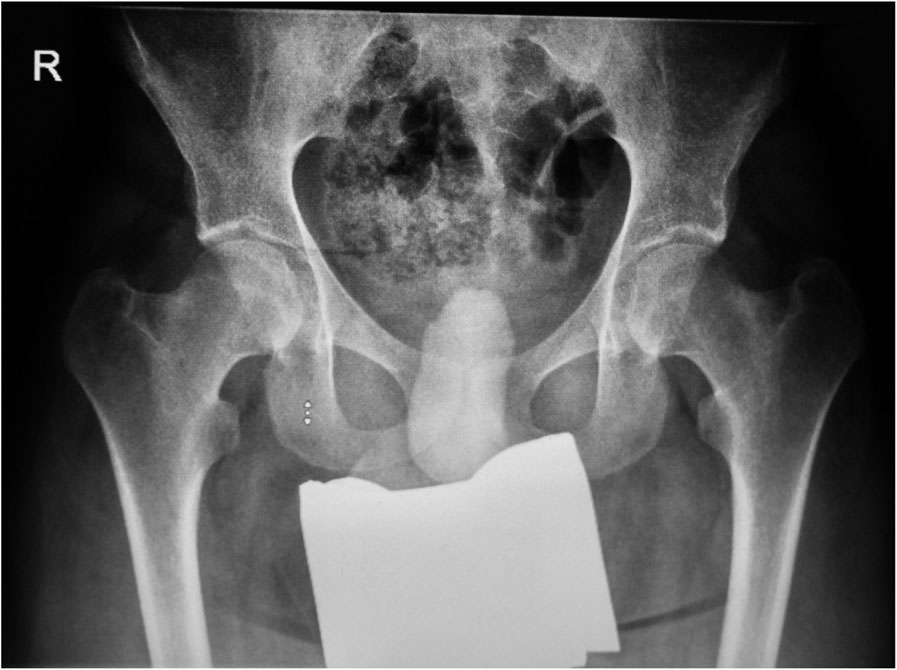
A 20-year-old MPS IIIA patient with an intermediate phenotype and physiological coverage of the femoral head. The neck of the femur is short and in a varus position. There are no radiographic signs of osteonecrosis of the femoral head
